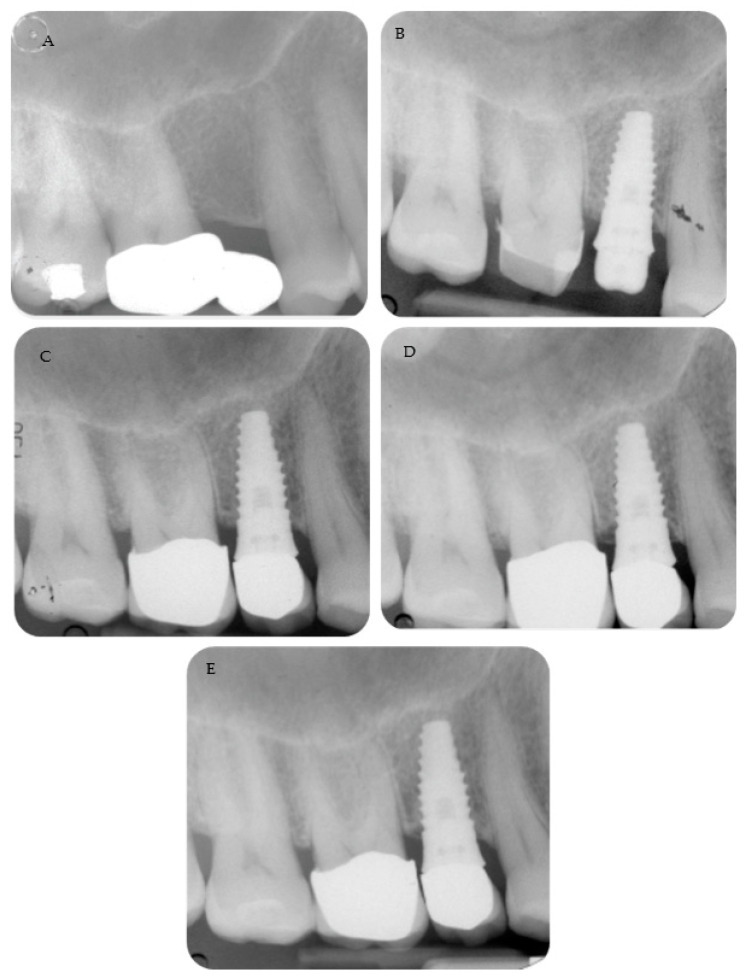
A 4.6 mm tissue-level implant was placed with a flapless approach. (A) Pre-operative radiograph. (B) Periapical radiograph shows the abutment screwed and the cemented provisional crown. Follow-up at 3 months. (C) Follow-up at 12 months with the metal ceramic crown cemented on the abutment. MBLs were stable at (D) 36 months and (E) 48 months, with limited bone loss.
